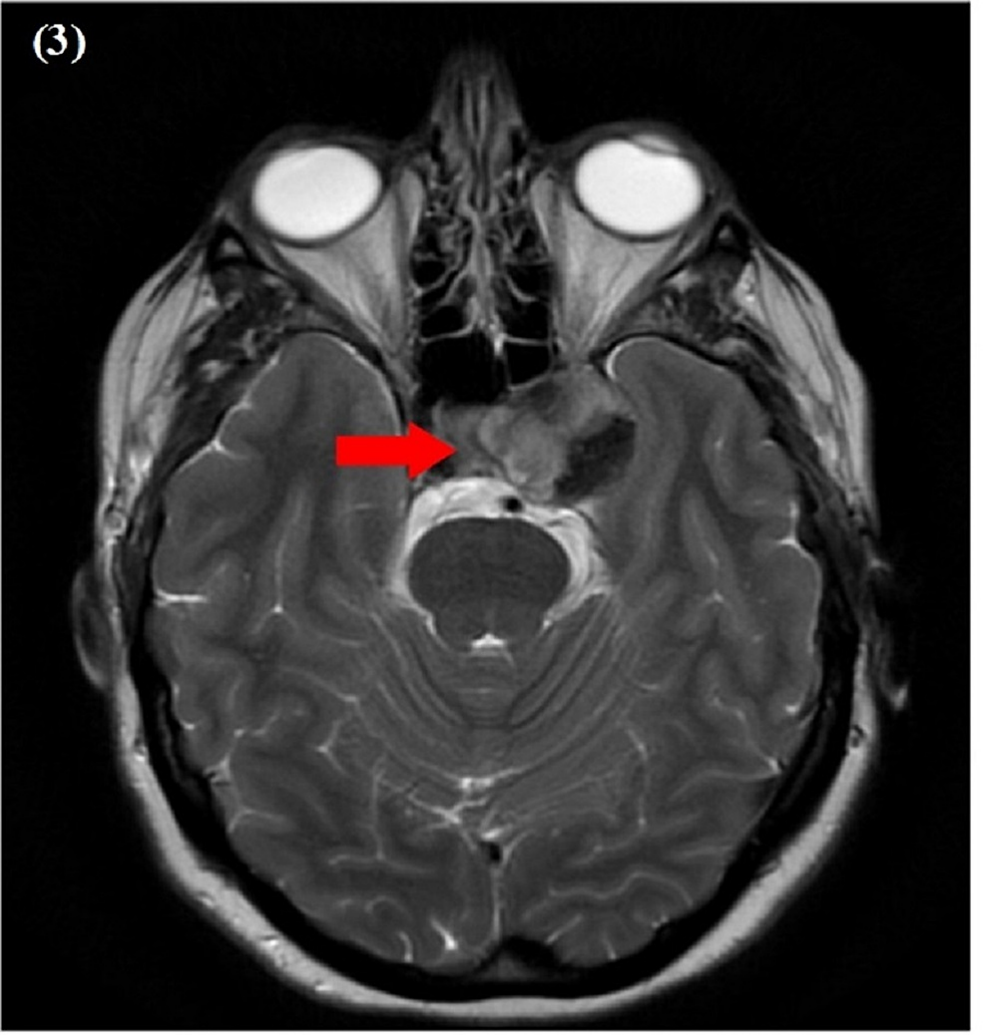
MRI brain with and without contrast: arrow showing an extra-axial tumor, measuring 3.3 x 2.4 x 3.0 cm, at the level of the left cavernous sinus with evidence of concavity involving the pituitary gland
